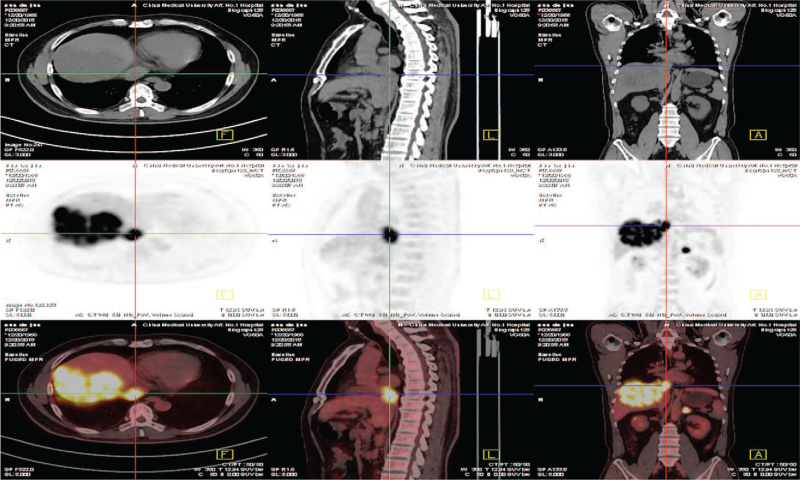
PET-CT showed the high FDG of the mass tumor in the right lobe of liver, the tumor thrombus in the inferior vena cava; the tumor thrombus in the right atrial and left adrenal gland metastasis. FDG = fluorodeoxyglucose, PET-CT = positron emission tomography-CT.
